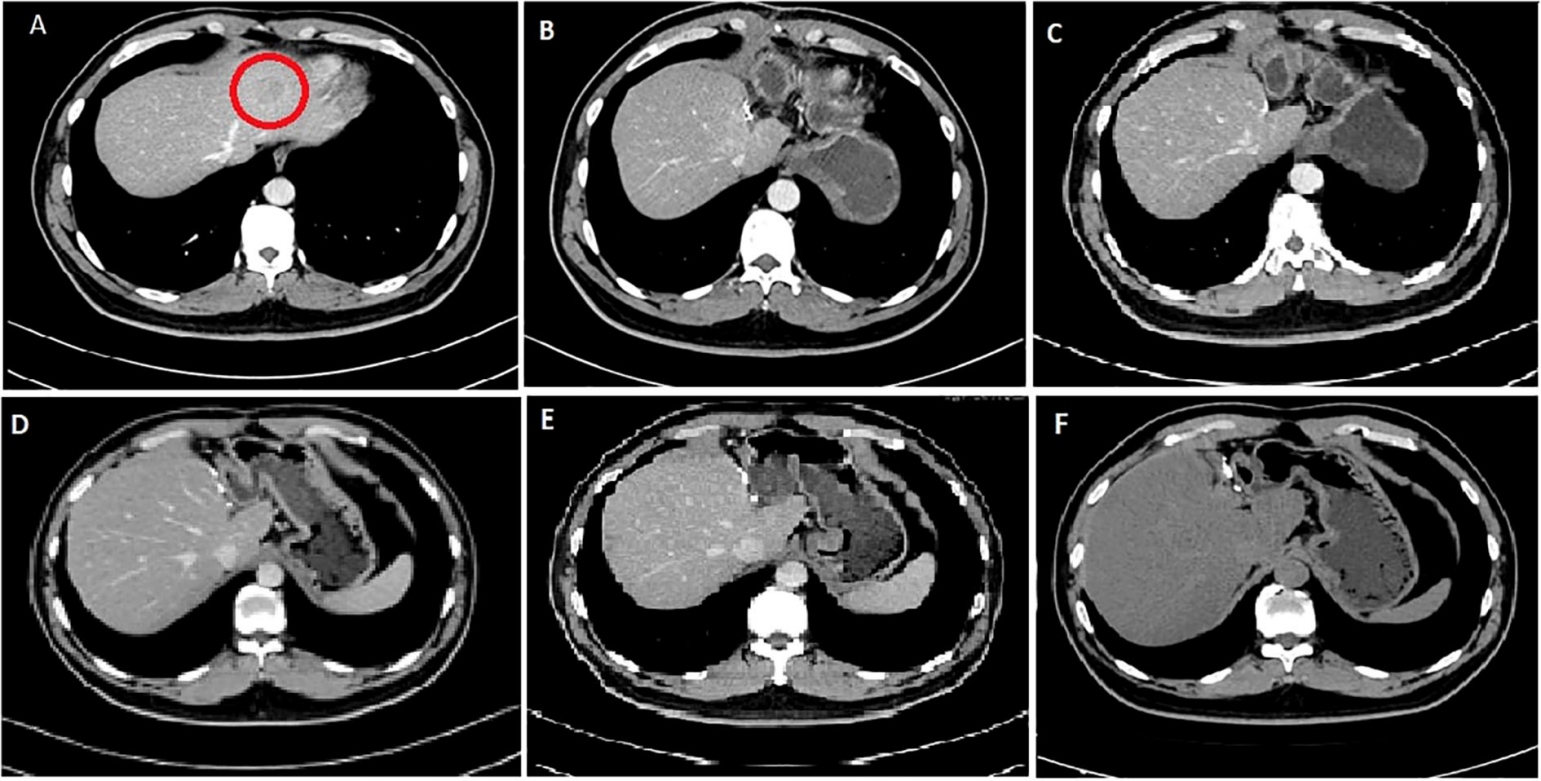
CT images of the 41-year-old male patient. (A) First diagnostic CT image showing tumor lesion (red circle) of size 3.0 × 2.8 cm taken in 2015. (B) CT image post-operation and after the first cycle of the GS regimen; no signs of tumor recurrence or metastasis. (C) CT image after the second cycle of the GS regimen. (D) CT image after the fourth cycle of the GS regimen. (E) CT image after the sixth cycle of the GS regimen. (F) CT image taken on follow-up in 2022.
